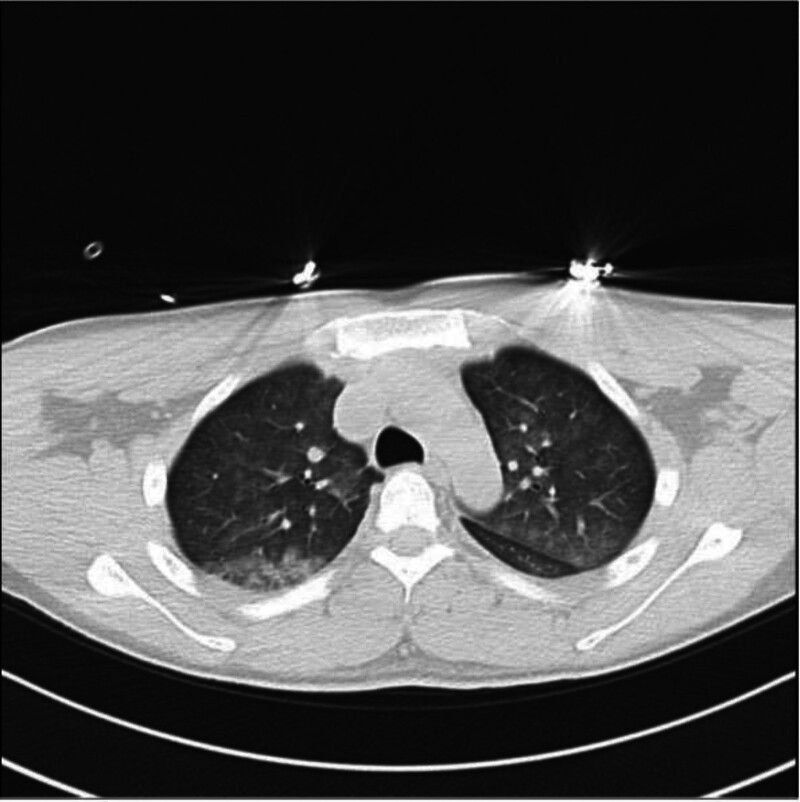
Follow-up chest CT (16 May, 2025): marked interval resolution of pulmonary edema 17 hours postoperatively. CT = computed tomography.
